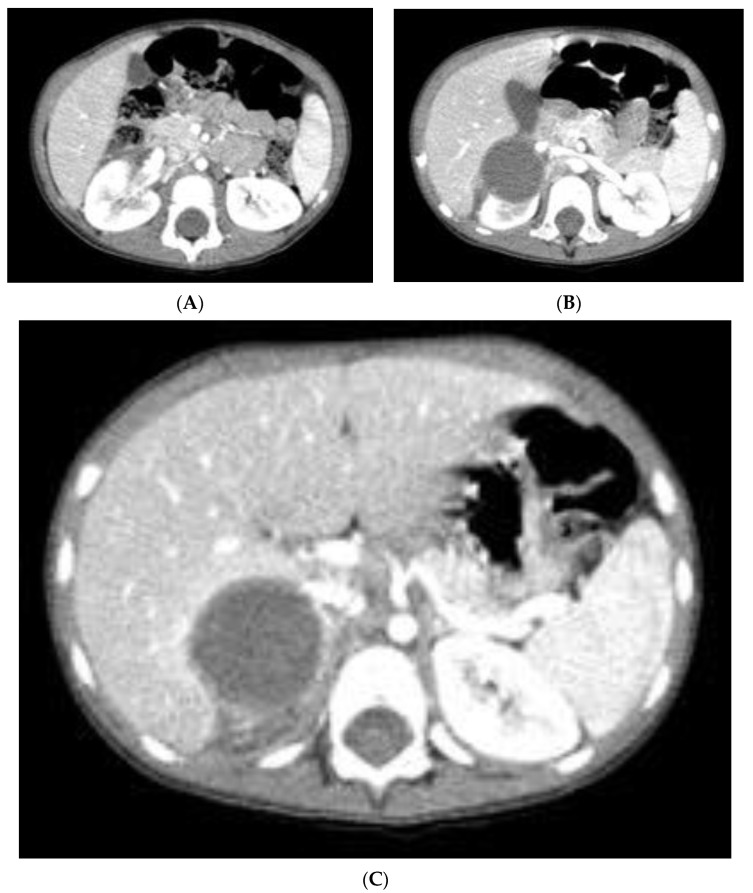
(A–C). Abdominal CT: (A): Transverse scan at the renal hylum, which is not involved by the tumor. (B): The mass impresses upon the caudal hepatic lobe without any signs of infiltration. (C): The mass indentates the right hepatic lobe, displaying a visible tumor pseudocapsule and a discernible cleavage plane with the liver.
